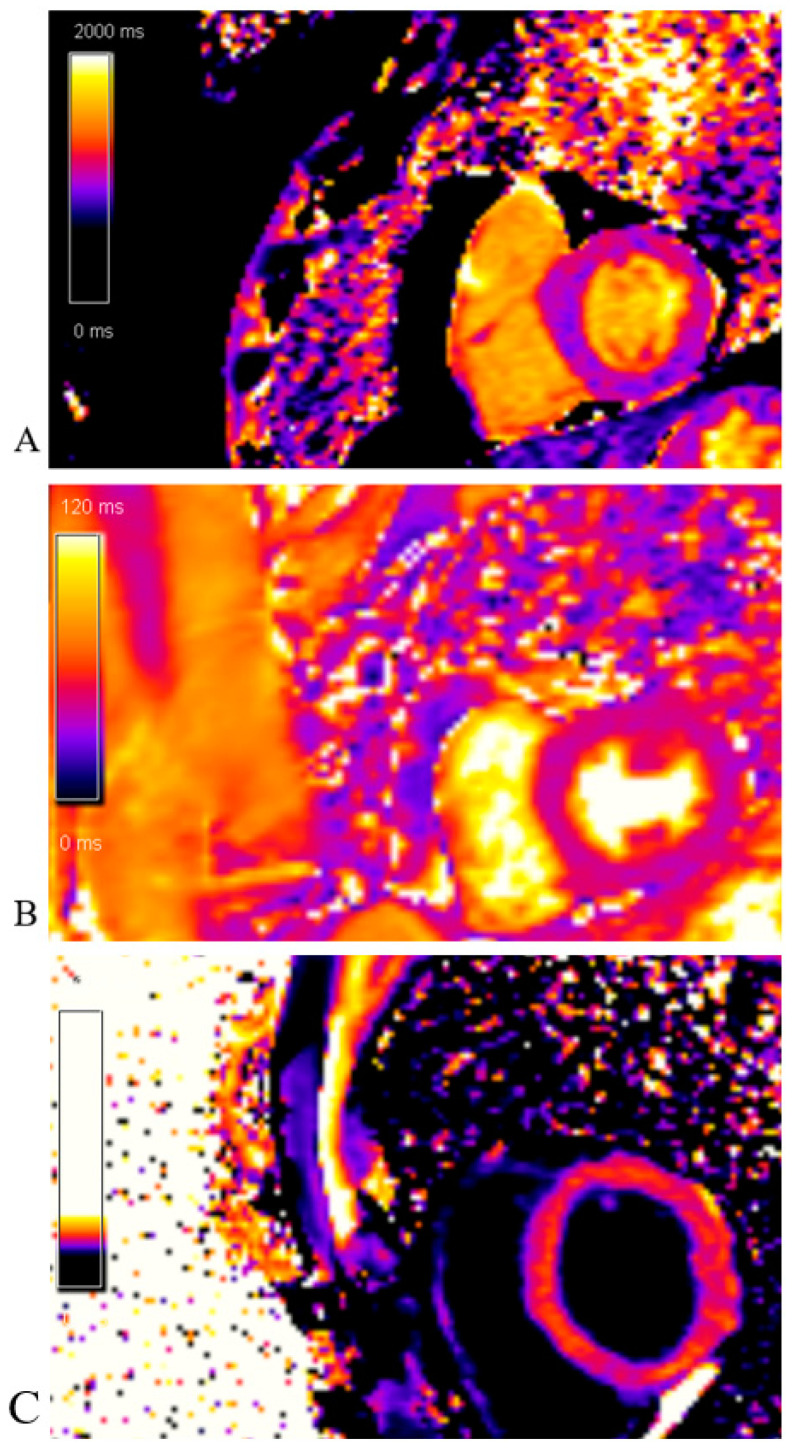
Cardiac magnetic resonance imaging: (A)Native T1 mapping sequence; without areas of prolonged native T1 time (edema/fibrosis); (B) T2 mapping sequence; without areas of prolonged T2 time (edema); (C) Post-contrast T1 mapping sequence; without areas of shortened post-contrast T1 time (fibrosis).
